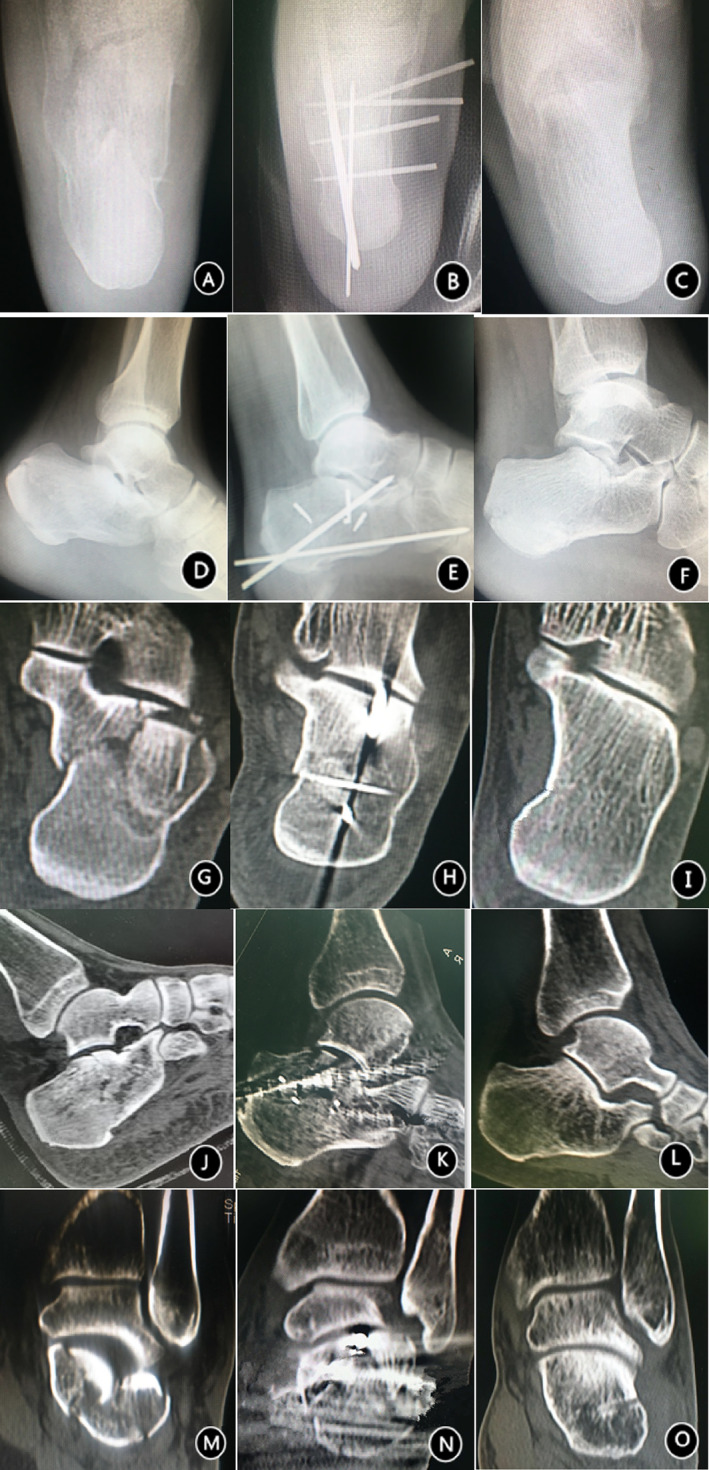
Patients were followed up with minimally invasive techniques. A 38‐year‐old man with a comminuted Sanders type IIIAB left calcaneal fracture caused by falling off a ladder was treated with orthopedic forceps and minimally invasive reduction with percutaneous headless compression screw internal fixation. The final follow‐up was at 33.2 months. (A) Axial X‐ray showing that the calcaneus was shortened and widened, with angled inversion. (B) After reduction, the calcaneal length, width, and inversion were corrected, and the headless compression screw on the inside was fixed and stable. (C) Good recovery of the calcaneus at the last follow‐up. (D) Lateral X‐ray showing collapse of the articular surface, a decrease in the Bohler angle such that it became negative, and a decrease in the height of the calcaneus. (E) Postoperative X‐ray showing recovery of the calcaneal height, return of the Bohler angle to within the normal range, and reliable headless compression screw fixation. (F) Normal calcaneal morphology at the last follow‐up. (G) Coronal CT showing calcaneal inversion and shortening, significantly calcaneal widening, and collapse and displacement of the articular surface. (H) Percutaneous reduction using orthopedic forceps restored the length of the calcaneus, corrected the inversion, and restored the articular surface. (I) At the last follow‐up, there was no loss of calcaneal length or varus, and the articular surface was flat. (J) Preoperative sagittal CT showing collapse and compression of the posterior calcaneal articular surface. (K) Prying with a Kirschner wire to restore the posterior calcaneal articular surface. (L) Flattening of the posterior calcaneal articular surface at the last follow‐up. (M) Preoperative CT showing displacement of the subtalar articular surface. (N) Smooth restoration of the subtalar articular surface and headless compression screw fixation. (O) Stable subtalar articular surface at the last follow‐up, with no traumatic arthritis.
